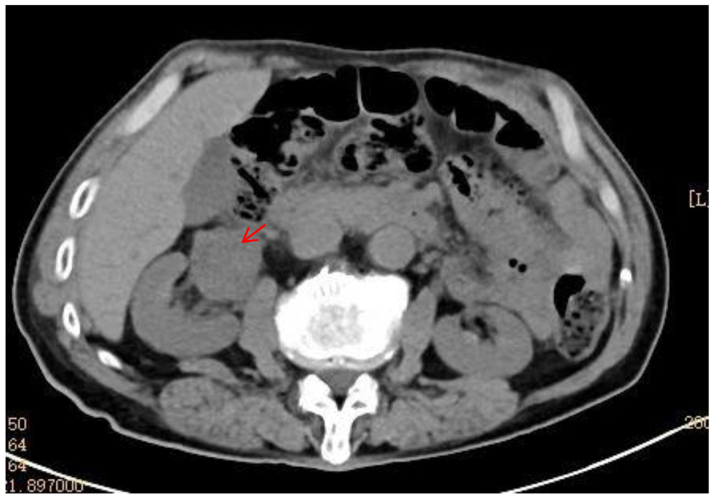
CT scan of the abdomen showing a tumor in the right renal pelvis. CT = computed tomography.
